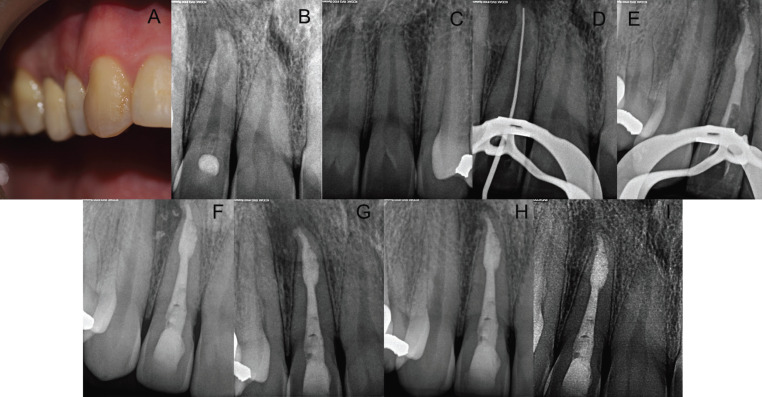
A. Clinical photography of the maxillary right lateral incisor. B. Initial radiograph of the Maxillary right lateral incisor; C. Maxillary left lateral incisor; D. Working length X-ray determination; E. MTA apical plug; F. Final restoration; G. Radiographic follow-up of tooth #7 at 5 months; H. At 2 years; I. At 3 years.
